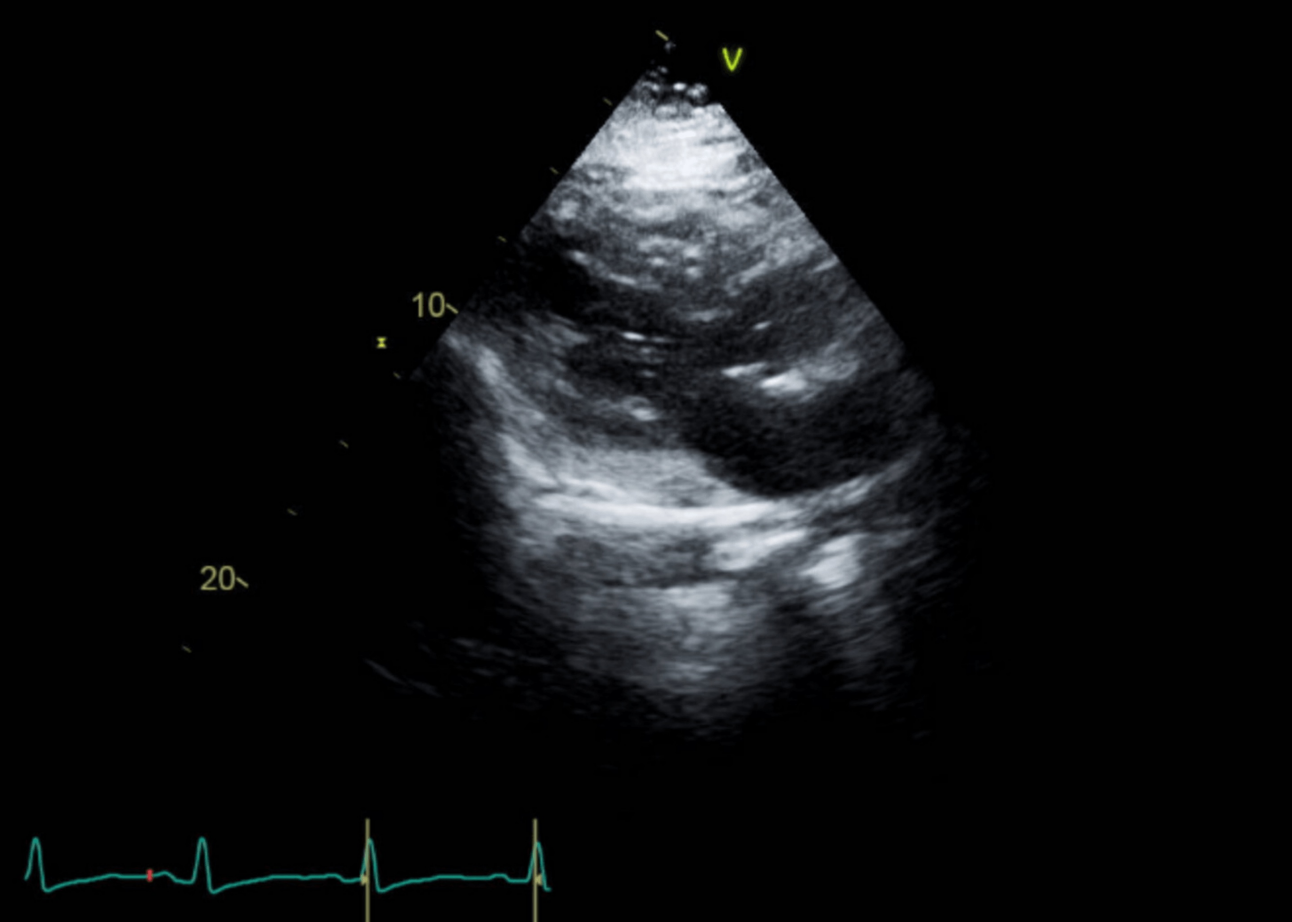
Parasternal long-axis view echocardiogram showing mild mitral valve leaflet thickening and tricuspid regurgitation.
